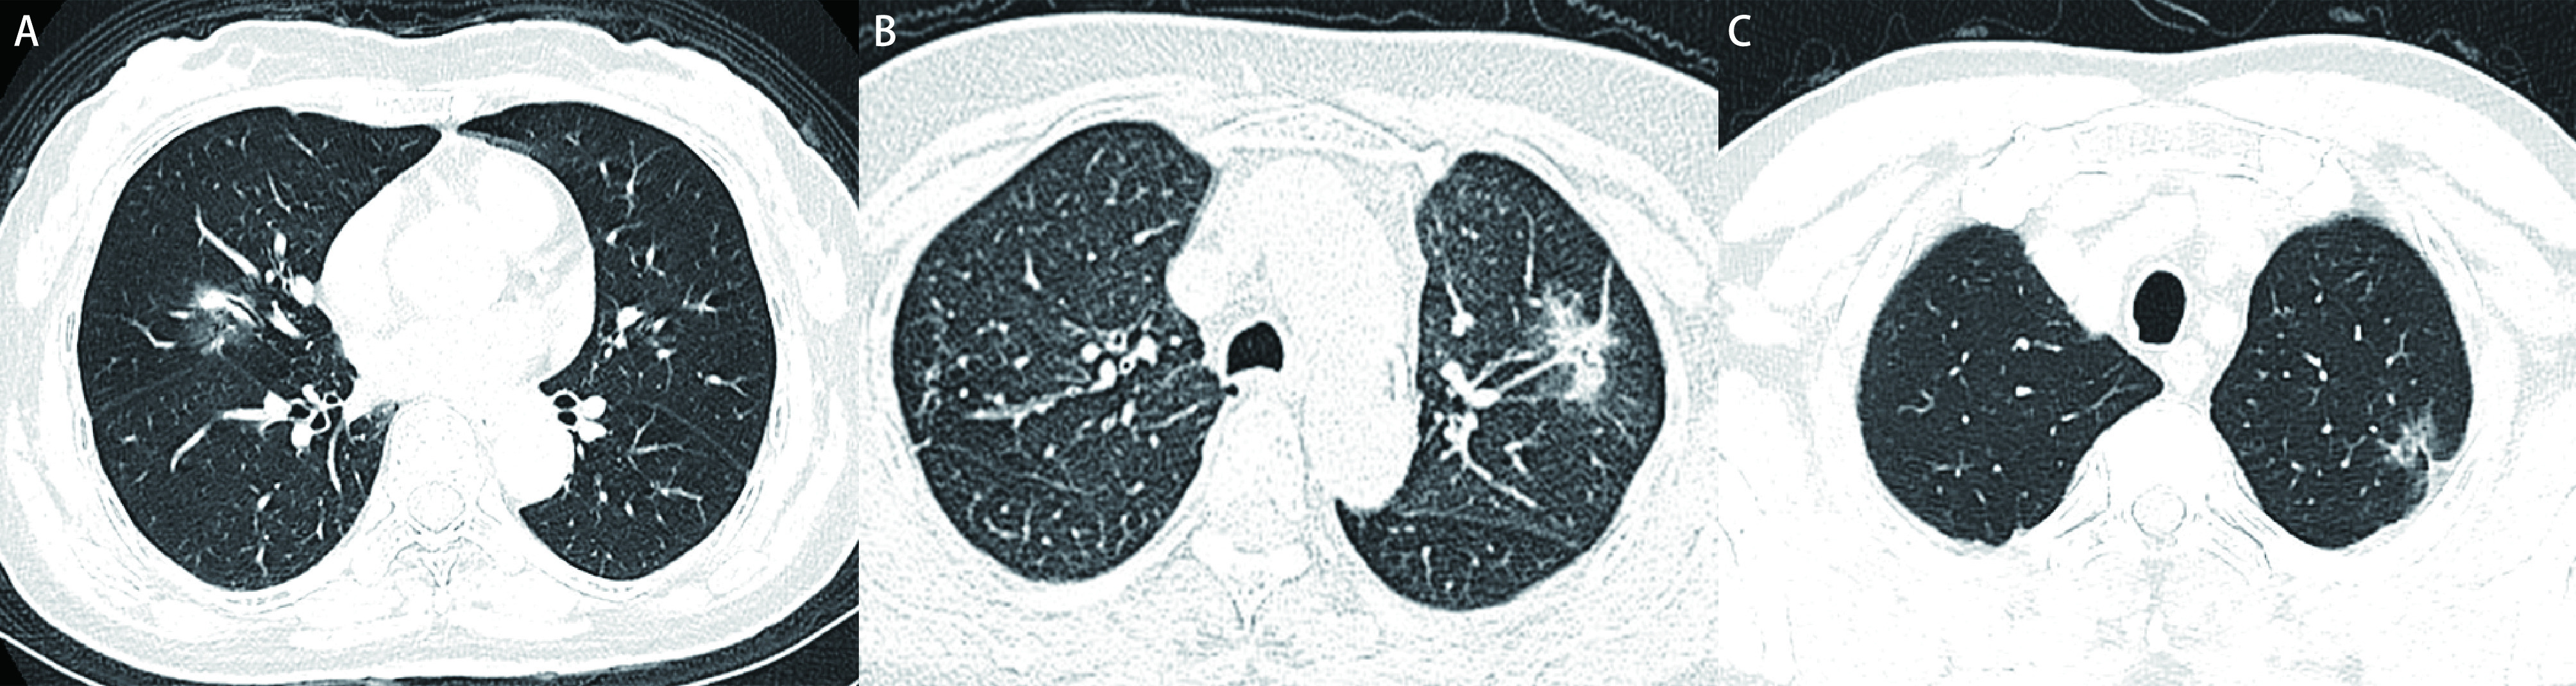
常见的肺癌相关影像学特征。A：支气管充气征；B：血管集束征；C：胸膜牵拉征 Common radiographic features associated with lung cancer. A: Air bronchogram; B: Vessel convergence sign; C: Pleural indentation.
